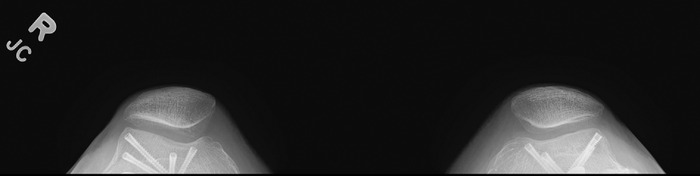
Merchant view radiograph shows postoperative alignment of both knees following bilateral trochleoplasty procedures.
